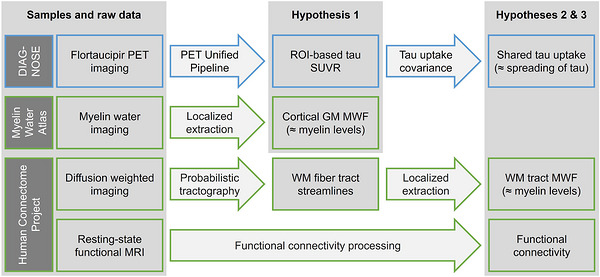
Overview of study samples, raw data, processing steps, and analyses. We used data on former American football players at risk for chronic traumatic encephalopathy as well as controls from the DIAGNOSE CTE research project (blue frames). We also used data from healthy young adult individuals from the Myelin Water Atlas and the Human Connectome Project (green frames). DIAGNOSE CTE, Diagnostics, Imaging, And Genetics Network for the Objective Study and Evaluation of Chronic Traumatic Encephalopathy; GM, gray matter; MRI, magnetic resonance imaging; MWF, myelin water fraction; PET, positron emission tomography; ROI, region of interest; SUVR, standardized uptake value ratio; WM, white matter
